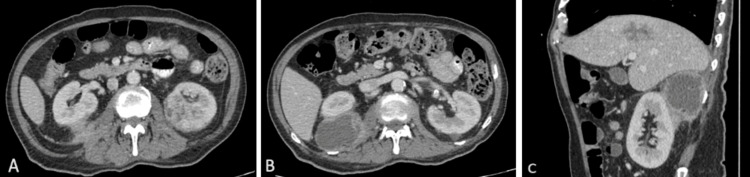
Axial and sagittal CT images: (A) Ill-defined hypodense lesions in both kidneys; (B-C) a large, rim-enhancing right perinephric collection with areas of liquefaction.
